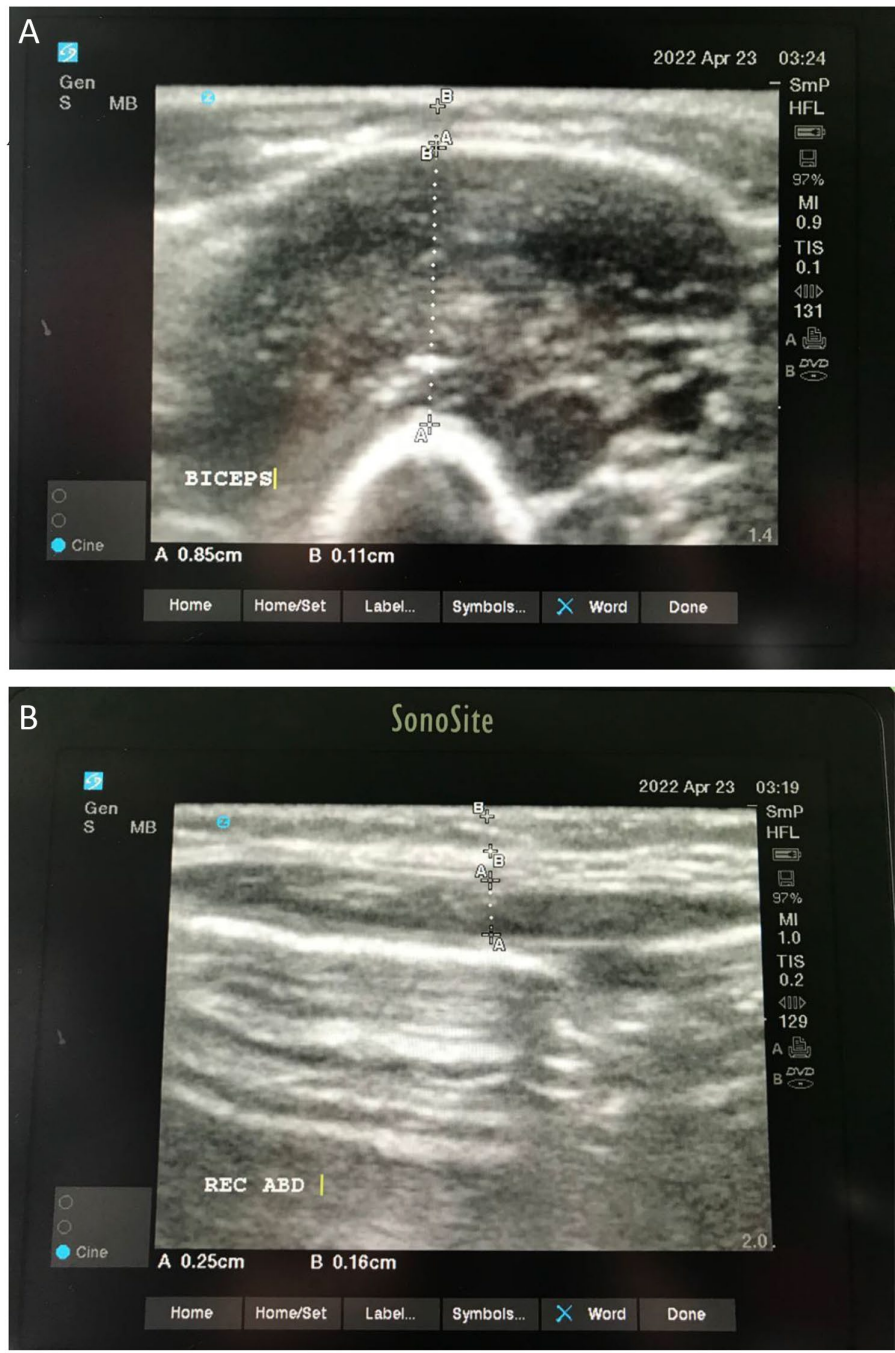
Ultrasound of (A) biceps brachialis; (B) rectus abdominis
